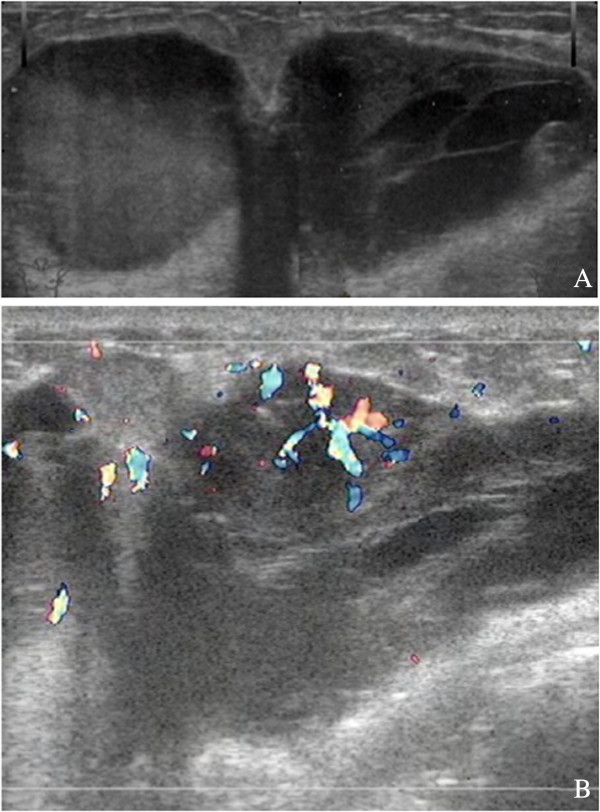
A 46-year-old woman with palpable masses in the left breast. A. Ultrasound demonstrates a pear-shaped complex echoic lesion with relatively indistinct margins. B. A hyperechoic area with abundant vessels within the mass. Another mass of the lesion is hyperechoic with spotted blood flow.
